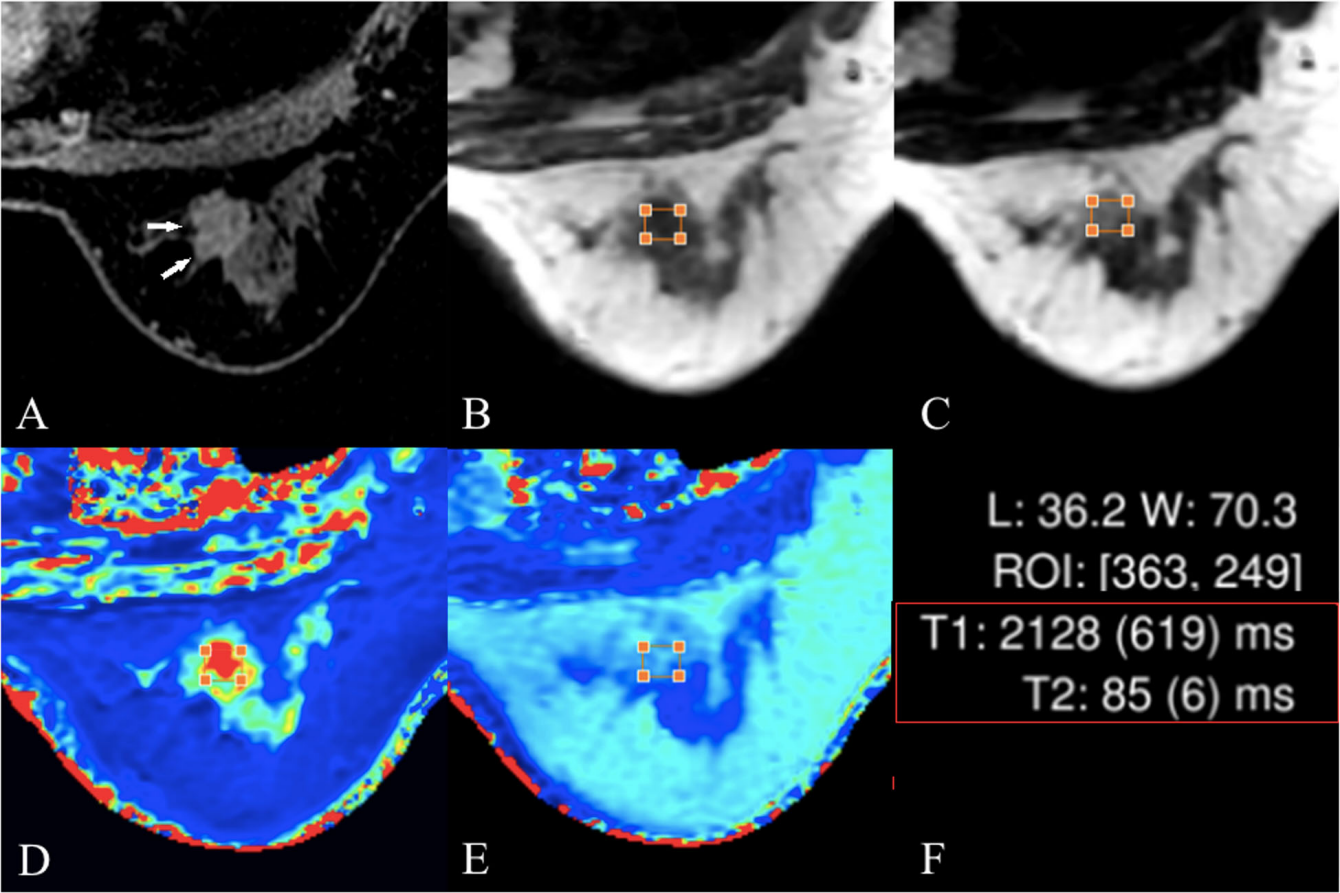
Female, 42 years old, invasive ductal cancer. a the lesion is shown using white arrows in the perfusion-enhanced image; b and c, T1- and T2-weighted images obtained from SyMRI respectively; d and e, T1 and T2 maps, respectively. The T1 and T2 values are shown in f
